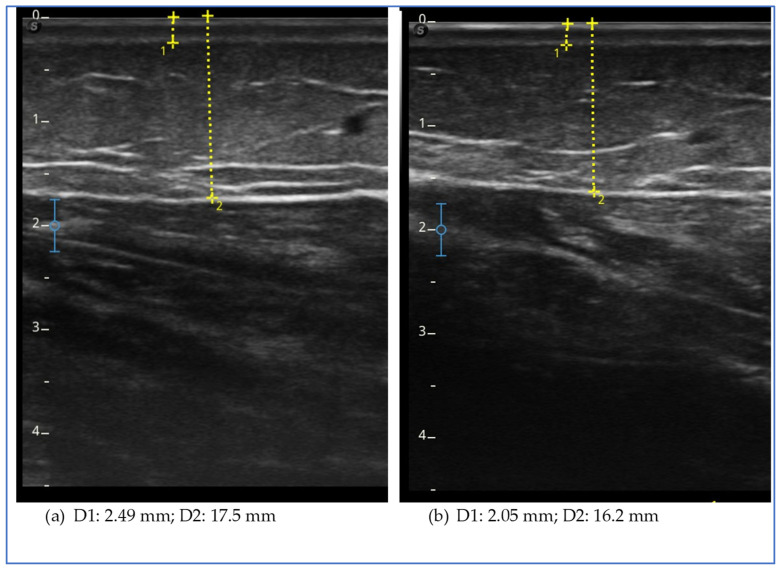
Ultrasound measurements for case report 2.
